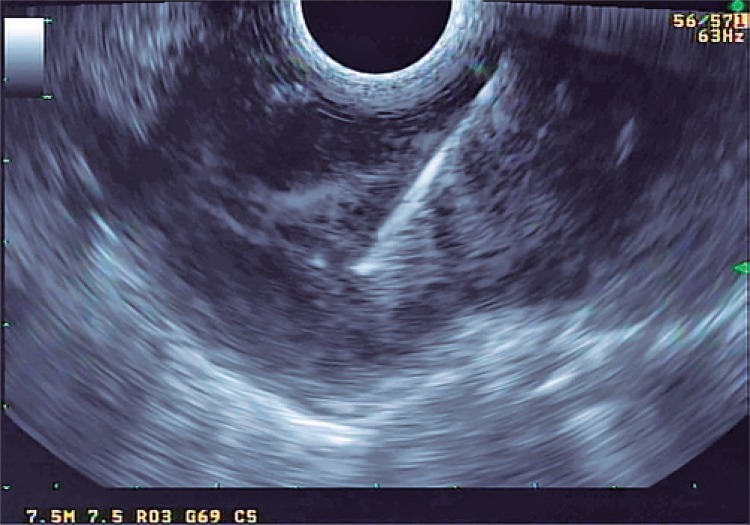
Ultrasound-guided aspiration puncture of a hypoechoic lesion of great proportions
